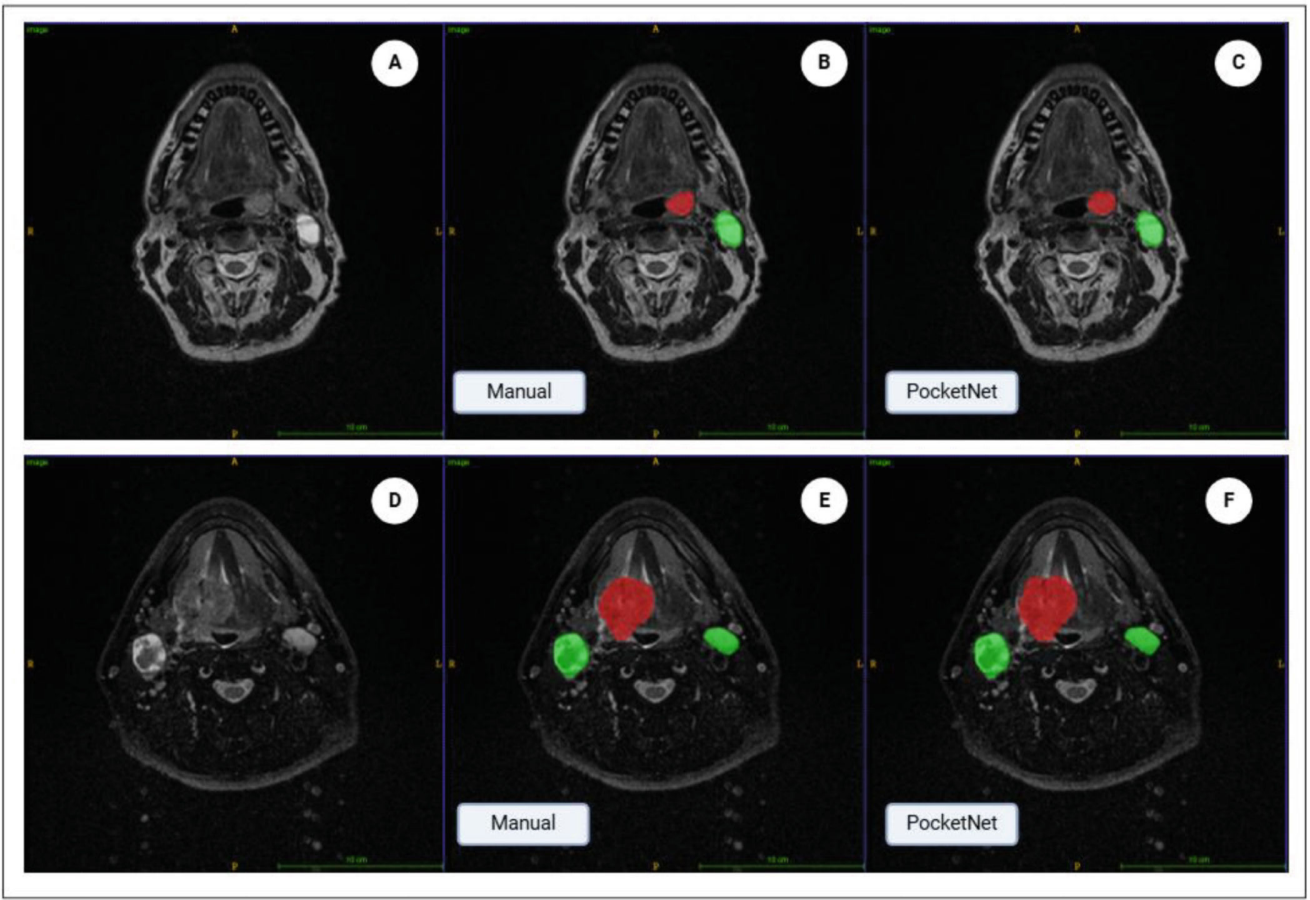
Manual and automated segmentation in two patients. A) Axial MRI of Case 1. B) Case 1 manual segmentation of GTVp (red) and GTVn (green). C) Case 1 Automated segmentation of GTVp (red) and GTVn (green). D) Axial MRI of Case 2. E) Case 2 manual segmentation of GTVp (red) and GTVn (green). F) Case 2 Automated segmentation of GTVp (red) and GTVn (green). The manual and automated tumor contours demonstrate good agreement (GTVp DSC = 0.88, 0.90 and GTVn DSC = 0.91,0.896).\CFO{}
